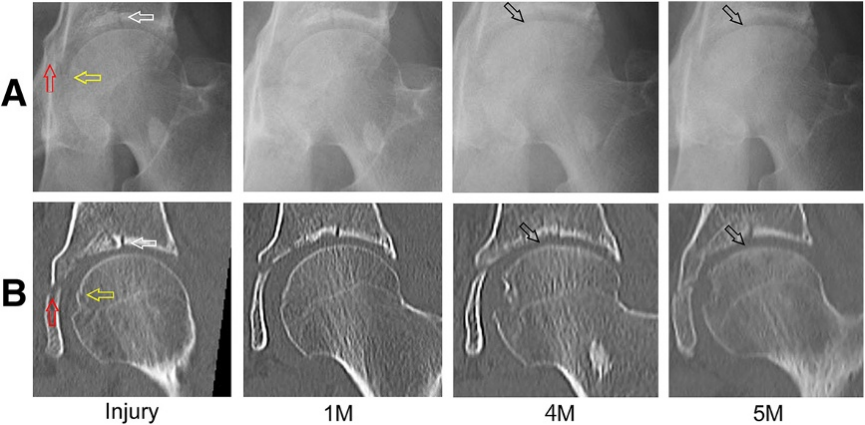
The weight-bearing area of the femoral head shows progressive collapse. (A) Anteroposterior plain radiographs and (B) coronal computed tomography (CT) images of the left hip at the time of injury (Injury) show a fracture in the acetabular roof (white arrow), inner wall of the acetabulum (red arrow), and medial articular surface of the femoral head (yellow arrow). The series of images (one month (1M), four months (4M) and five months (5M) after injury) show the progression of collapse in the femoral head (black arrows), with residual displacement of the fracture in the acetabular roof.
